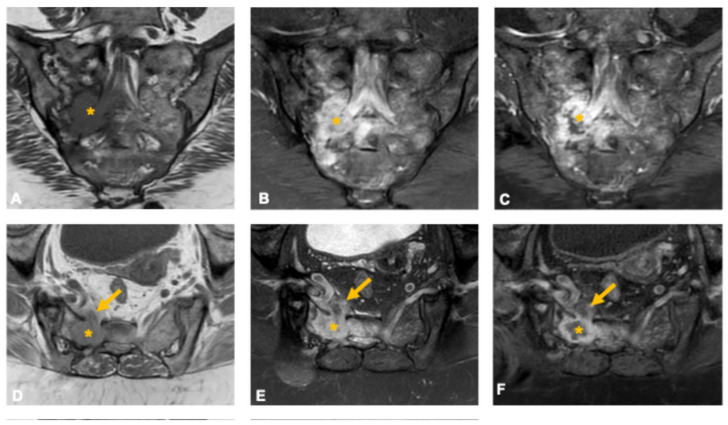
Paracoccidioidomycosis bone lesion in a 54-year-old woman. Coronal and axial MR images (A–F) show a destructive lesion centered in the right sacral ala (asterisk) with intermediate signal intensity on T1-weighted imaging (A,D), intermediate-to-high signal on T2-weighted Imaging (B,E) and heterogeneous enhancement with a central hypoenhancing area (C,F). Perilesional bone edema is noted, with extension of the edema/inflammatory process into the right sacral foramen, involving the neural root (arrows).
